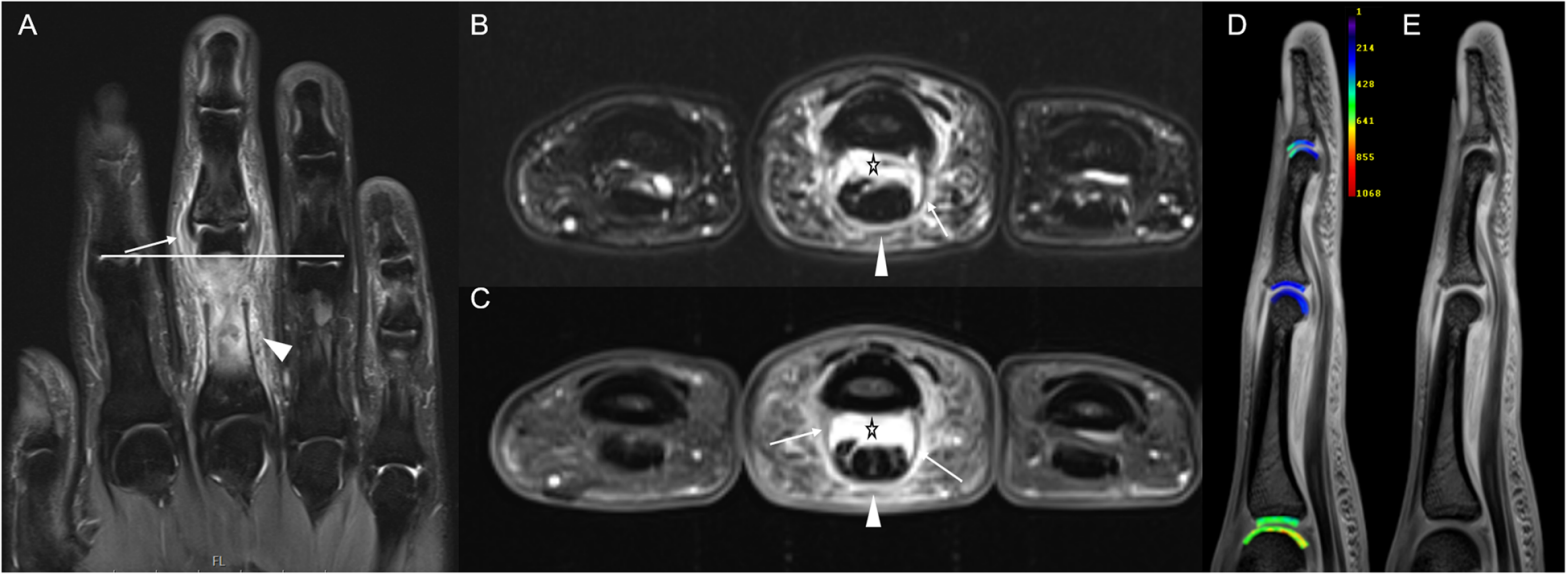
Right hand of a 26-year-old male with psoriatic arthritis (PsA; disease duration 39 months). Coronal STIR image (a) of digits 1–5, transversal fat-saturated (fs) T2-weighted image of digits 2–4 (b), and the corresponding transversal fs contrast-enhanced T1-weighted image (c) at the distal portion of the proximal phalanges. The horizontal white bar in a indicates the level of transversal slices (b, c). Sagittal fs proton density-weighted image of the third digit (d). a Increased signal at the collateral ligaments and synovitis of the proximal interphalangeal (PIP) joint of the third digit (white arrow). Periarticular inflammation around the PIP joint and the body of the proximal phalanx of the third digit (arrowhead). b, c Extensive flexor tenosynovitis (asterisk) and periarticular inflammation in the subcutaneous tissues (arrowhead) alongside thickened flexor tendon pulleys (arrow). d, e Representative sagittal T1-weighted images of the MCP, PIP, and DIP joints of the 3rd digit. Following iv contrast administration and appropriate delay of 40 min, a gives the morphological T1 map, while b gives the corresponding parameter map with dGEMRIC values [ms] overlaid. Note the significant decrease in dGEMRIC indices of the PIP joint as compared to the MCP joint. Also, decreased dGEMRIC indices of the volar aspect of the DIP joint
